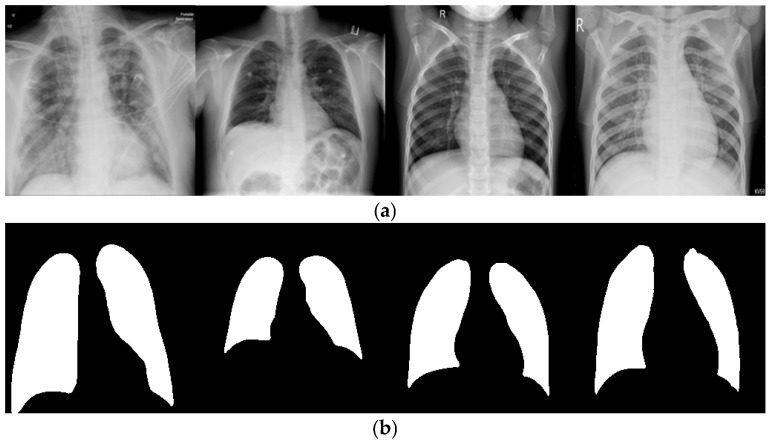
Example X-ray images from the dataset [14]. From left to right: COVID-19, lung opacity, normal, and viral pneumonia. (a) X-ray images and (b) corresponding mask images.
